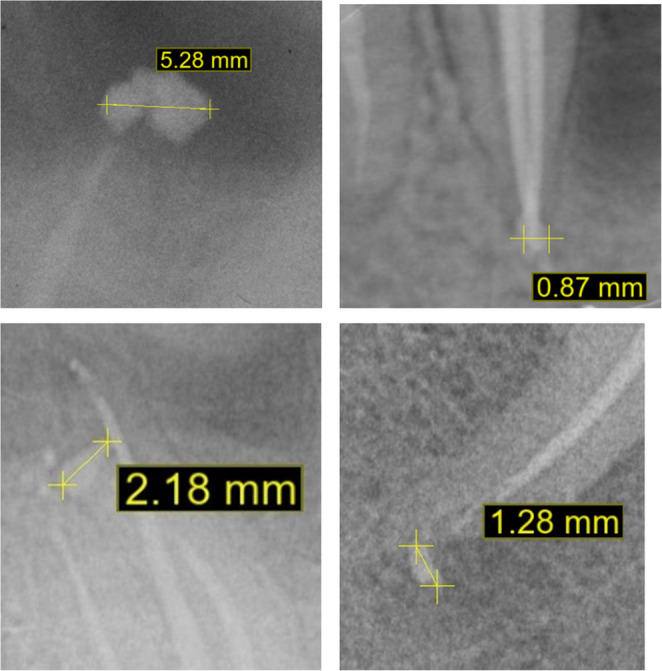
Examples of apical extrusion measurements using the digital software. The images revealed extrusions of different size (from 0.87 mm to over 5 mm) and shapes (linear, round shaped and irregular shaped morphologies), due to the presence or absence of periapical lesions
